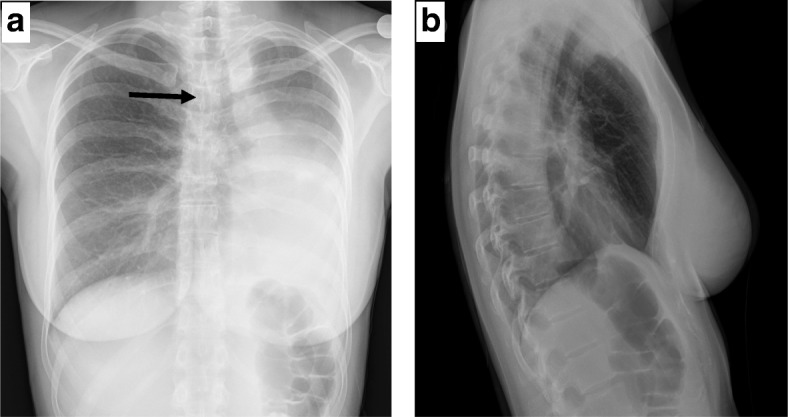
a. Chest radiograph (postero-anterior view) showing opacification of the left middle and lower lung zone. Tracheal deviation towards the left is also evident; (black arrow). b. Lateral chest radiograph
